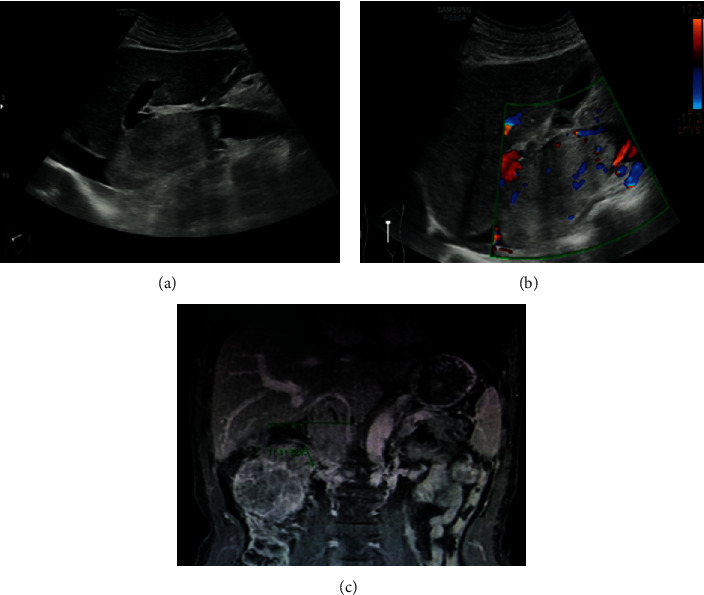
Imaging and pathological findings in a 63-year-old male with clear cell renal cell carcinoma (ccRCC) in the right kidney with inferior vena cava (IVC) tumor thrombus (IVCTT, Mayo III). (a) Ultrasound images showing that the IVC is completely obstructed and has no residual blood flow. (b) Coronal gadolinium enhanced T1-weighted image showing the IVCTT and its maximum coronal diameter of 37.5 mm. The possibility of IVC invasion was 68% in this case. In consideration of tumor invasion of IVC wall, so IVC resection was performed.
